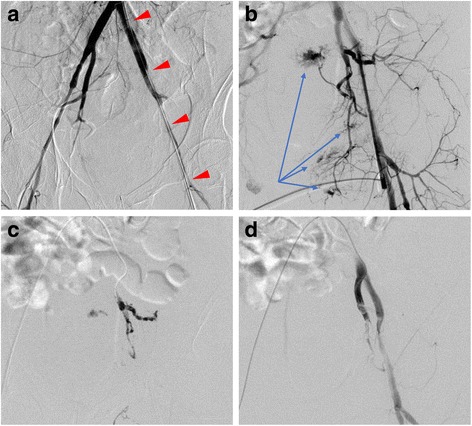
Transcatheter arterial embolization for the pelvic fracture. The contrast media extravasation ofthe internal iliac artery area is clear in computed tomography. First, the aortic occlusion catheter was inserted from the left femoral artery (arrowheads) and was embolized with a gelatin sponge from the origin portion of the bilateral internal iliac artery while the aorta was occluded at the first lumbar vertebra level. a Embolization of the lumbar artery and middle sacral artery was additionally performed and angiography from the sheath reinserted to the left femoral artery presented re-bleeding (arrow). b As it was difficult to arrest hemorrhage with a gelatin sponge, hemorrhage was arrested by embolizing additionally with n-butyl-2-cyanoacrylate (c and d)
